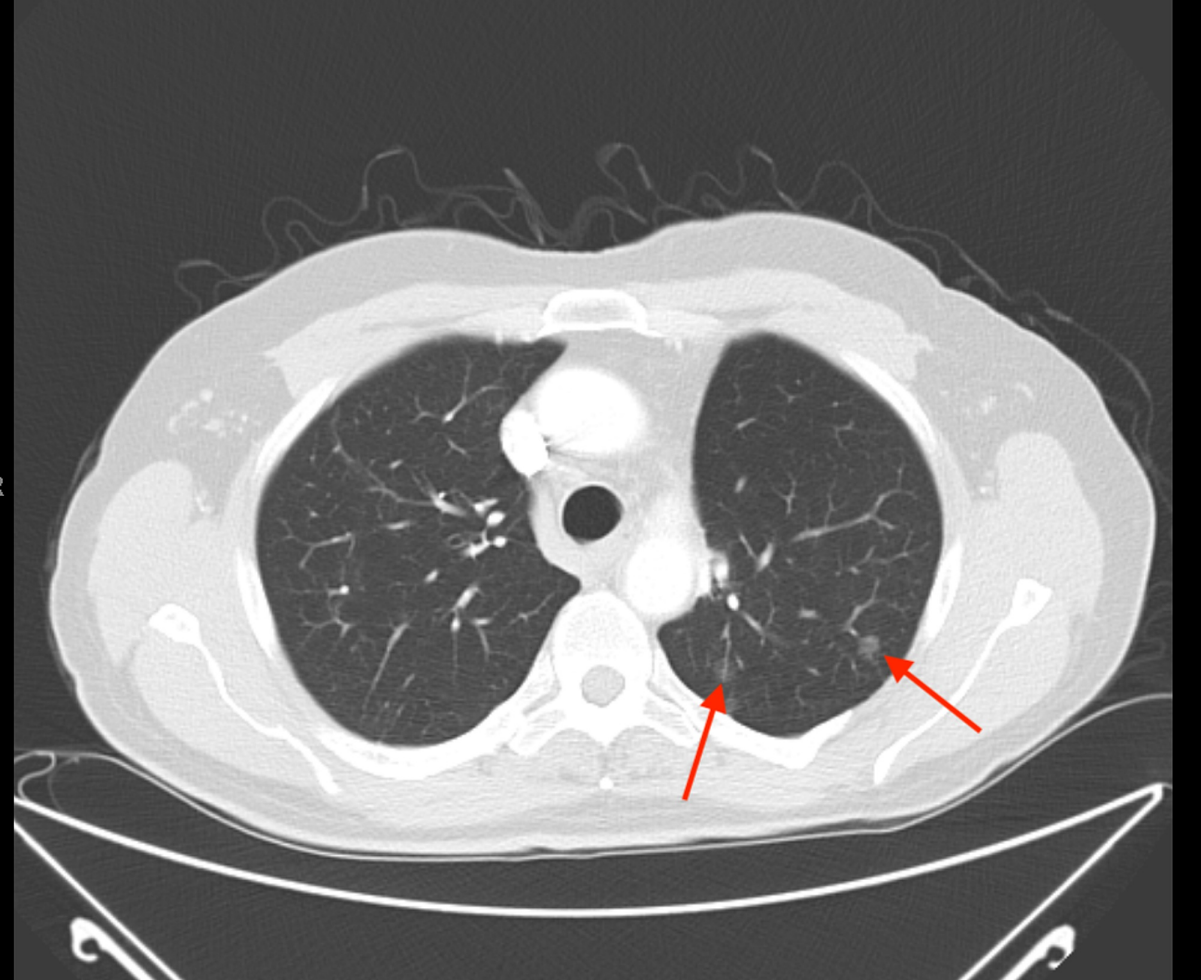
CT chest after chemotherapy showing the stable appearance of pulmonary nodules (red arrows) CT: computed tomography
